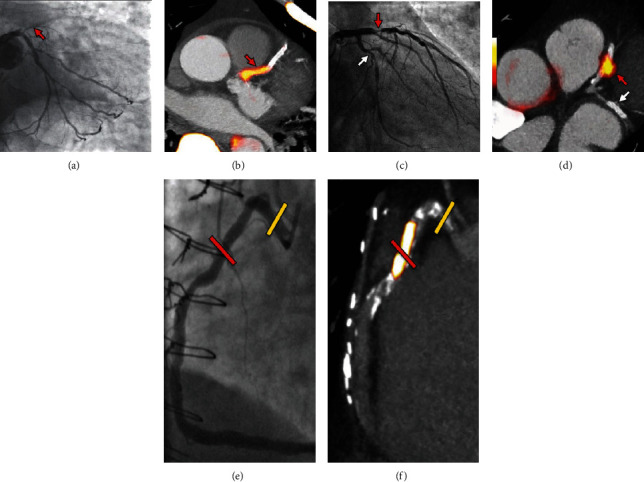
Focal ^18^F-NaF in patients with myocardial infarction and stable angina. A patient with acute ST-segment elevation myocardial infarction with (a) proximal occlusion (red arrow) of the left anterior descending artery on invasive coronary angiography and (b) intense focal ^18^F-NaF (tissue-to-background ratios, culprit 2.27 vs. reference segment 1.09 (108% increase)) uptake (yellow-red) at the site of the culprit plaque (red arrow) on the combined PET and CT. A patient with anterior non-ST-segment elevation MI with (c) culprit (red arrow; left anterior descending artery) and bystander nonculprit (white arrow; circumflex artery) lesions on invasive coronary angiography that were both stented during the index admission. Only the culprit lesion had increased ^18^F-NaF uptake (tissue-to-background ratios, culprit 2.03 vs. reference segment 1.08 (88% increase)) on PET-CT (d) after percutaneous coronary intervention. In a patient with stable angina with previous coronary artery bypass grafting, (e) invasive coronary angiography showed nonobstructive disease in the right coronary artery. (f) Corresponding PET-CT scan showed a region of increased ^18^F-NaF activity (positive lesion, red line) in the midright coronary artery (tissue-to-background ratio, 3.13) and a region without increased uptake in the proximal vessel (negative lesion, yellow line). This figure was originally published in the *Lancet* under the Creative Commons Attribution 4.0 International License (http://creativecommons.org/licenses/by/4.0/) (data from Joshi et al. [20]).
